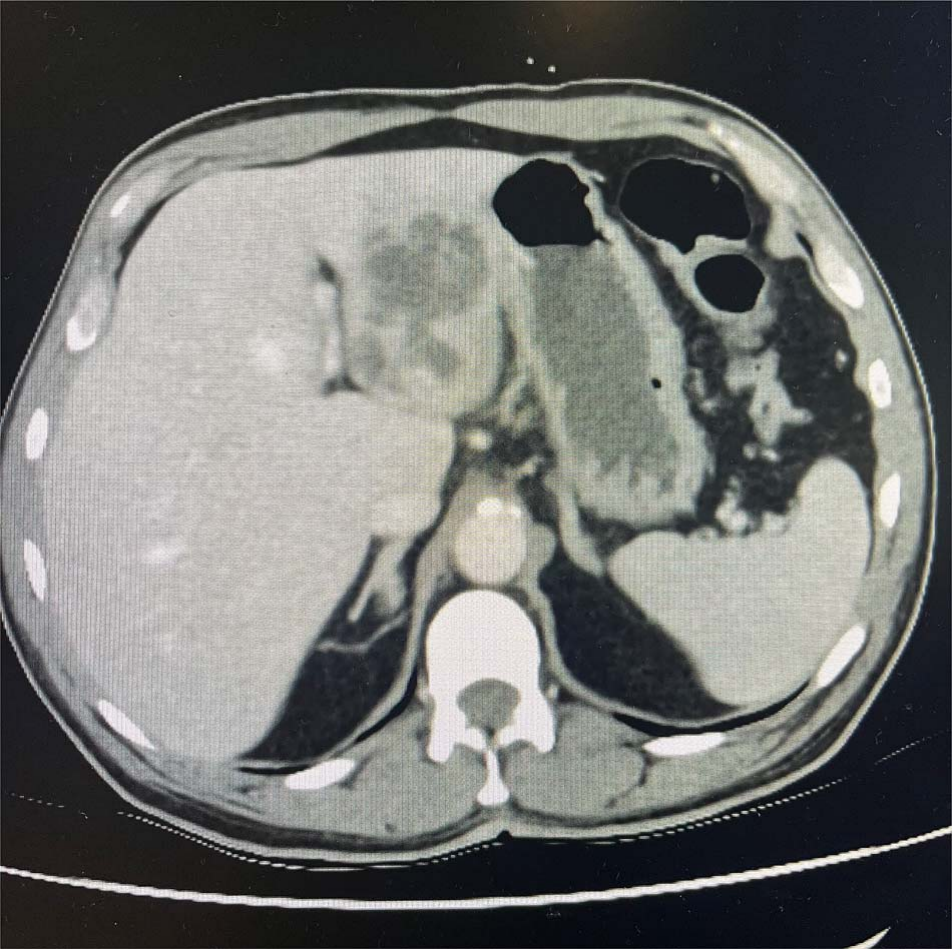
CT abdomen and pelvis in portal venous phase in axial slice. Hepatic abscess seen in left hepatic lobe.
